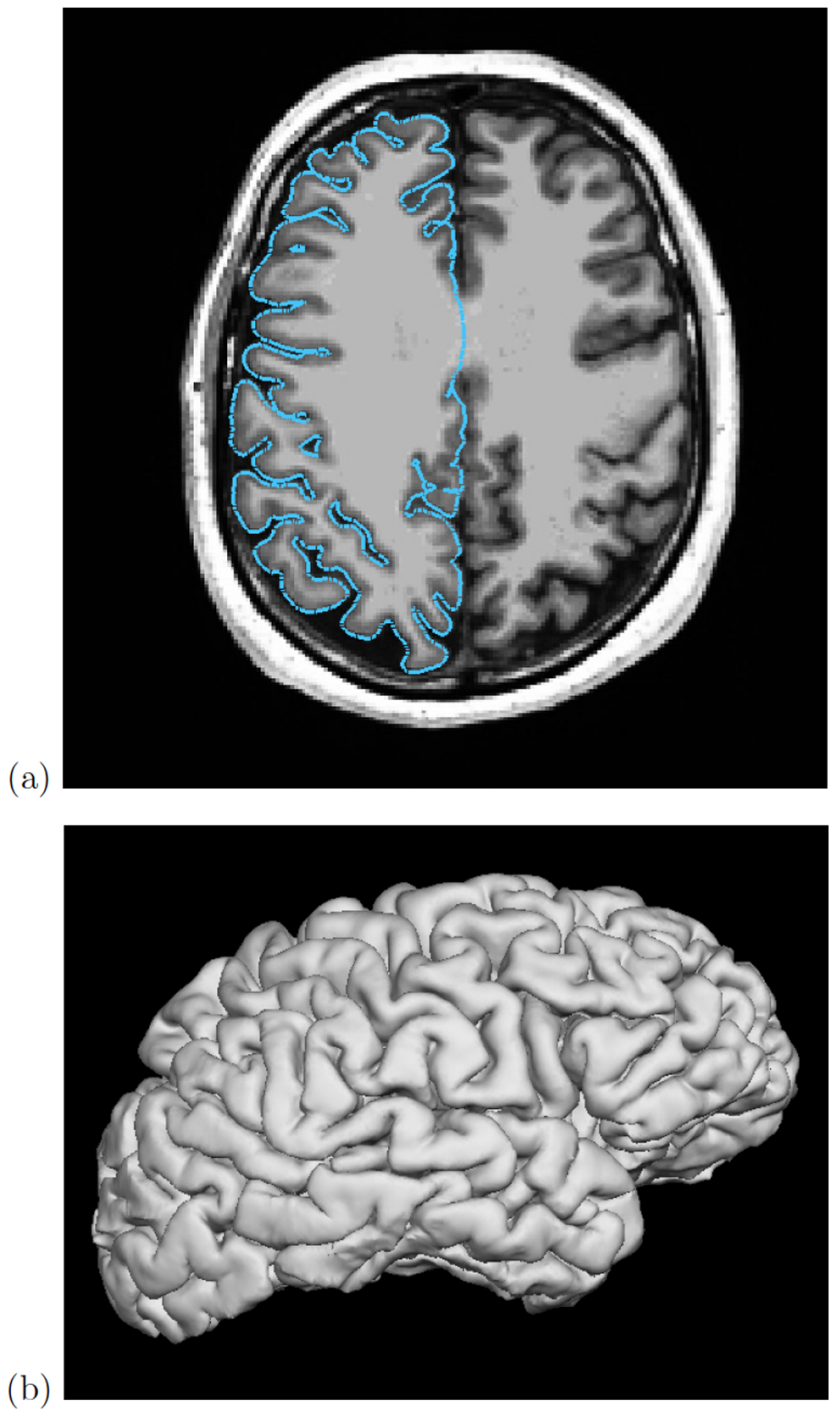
Pial surface renderings. (a) FreeSurfer tkmedit rendering of T1-weighted structural MR image and cross-section through extracted pial surface; and (b) FreeSurfer tksurfer 3-d rendering of the pial surface.
